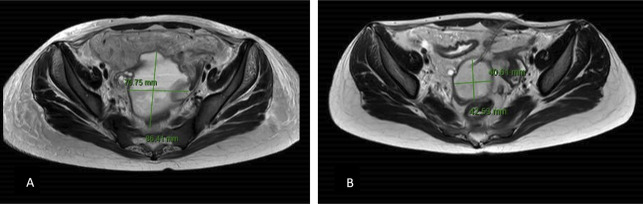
(A) Pelvic MRI showed a large pelvic fluid collection in the Douglas pouch measuring 7 × 9 × 4 cm (left-right × anterior-posterior × cranial-caudal), not separated from the sigmoid colon, associated with diffuse surrounding fat creeping and edema. (B) 1 week after the drain was inserted, showing a slight decrease in size. MRI, magnetic resonance imaging.
